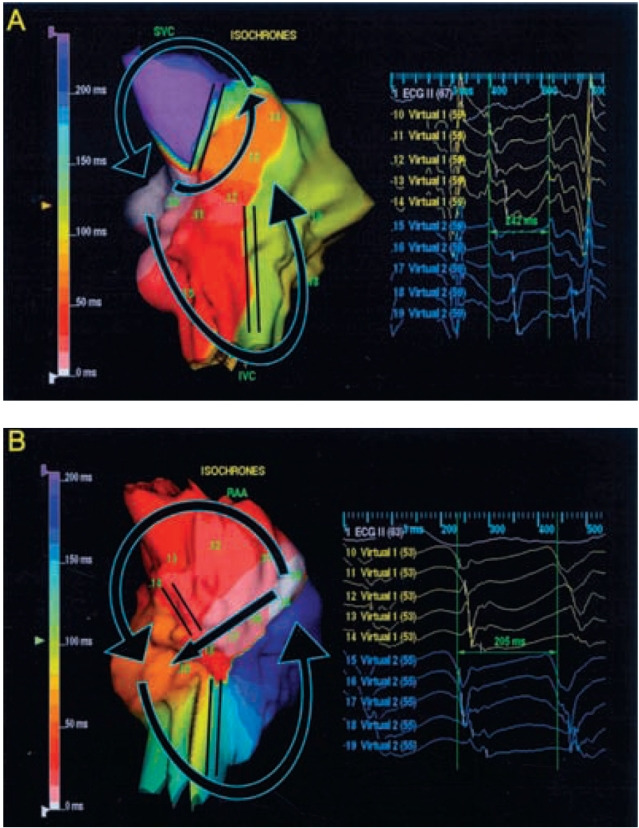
A: Isochronal map showing the reentrant circuit of double loop reentry, indicating CW typical atrial flutter and CW upper loop reentry, in the right posterior oblique view (black arrows). Note slow conduction through the CT gap with fractionated unipolar electrogram (virtual 12). Virtual 10 to 14 were located along the a conduction gal in the CT and virtuals 15 to 19 were located in the inferior right atrium involving the isthmus. B: Isochronal map showing the reentrant circuits of double loop reentry, including CW typical atrial flutter and lower loop reentry, in the right posterior oblique view (black arrows). Note slow conduction through the CT gap with fractionated unipolar electrogram (virtual 18). Virtual 10 to 14 were located in the superior lateral right atrium and virtual 15 to 19 were located along a conduction gap in the CT. CT = crista terminalis; CW = clockwise; IVC = inferior vena cava; SVC = superior vena cava. (Reproduced with permission from Tai CT, Huang JL, Lee PC, et al. High resolution mapping around the crista terminalis during typical atrial flutter: new insights into mechanisms. J Cardiovasc Electrophysiol 2004;15:406-414, Blackwell Publishing)
